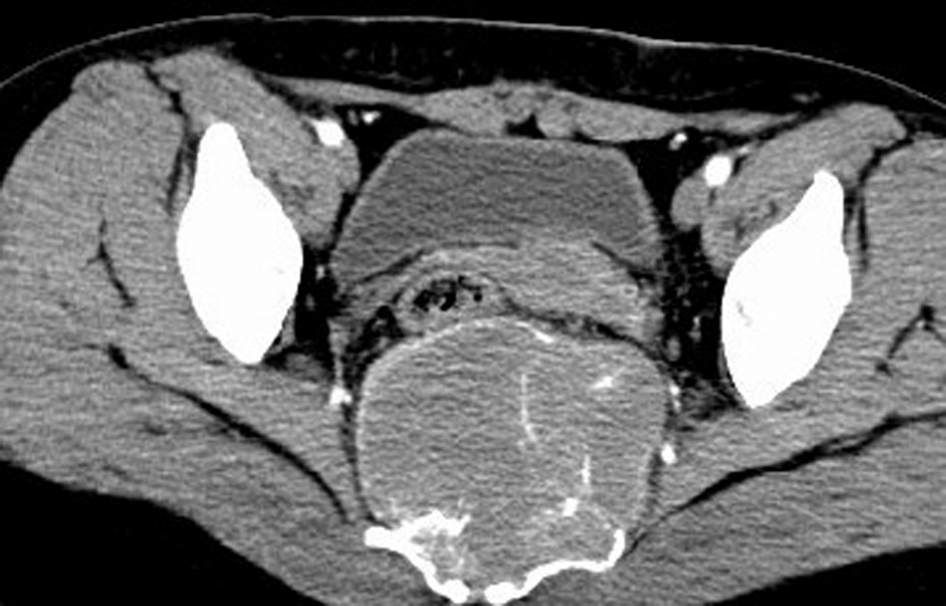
Contrast-enhanced axial CT shows a blood-riched, well defined, expansile, intraosseous tumor without cortical interruption in the sacrum. Some dense, coarse, trabeculated-like bones and residual bone crests within the tumor are observed. Extraosseous involvement of the soft tissue is absent.
